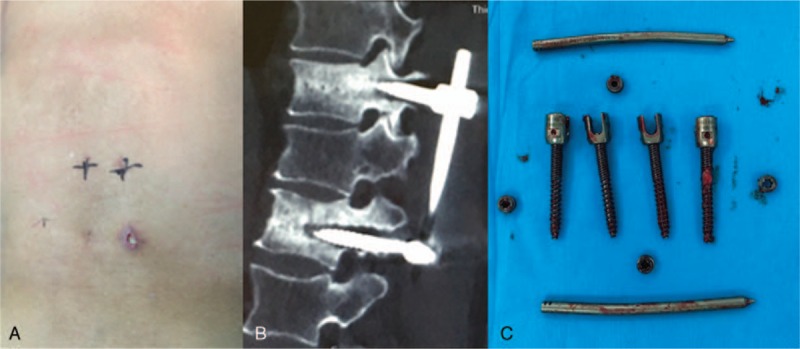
Female, 51 years old, T12 vertebral fracture (type A1): incision infection combined with sinus formation 10 months after operation (A); CT revealed fracture healing with screw loosening (B); removal of implants at revision surgery (C). CT = computed tomography.
